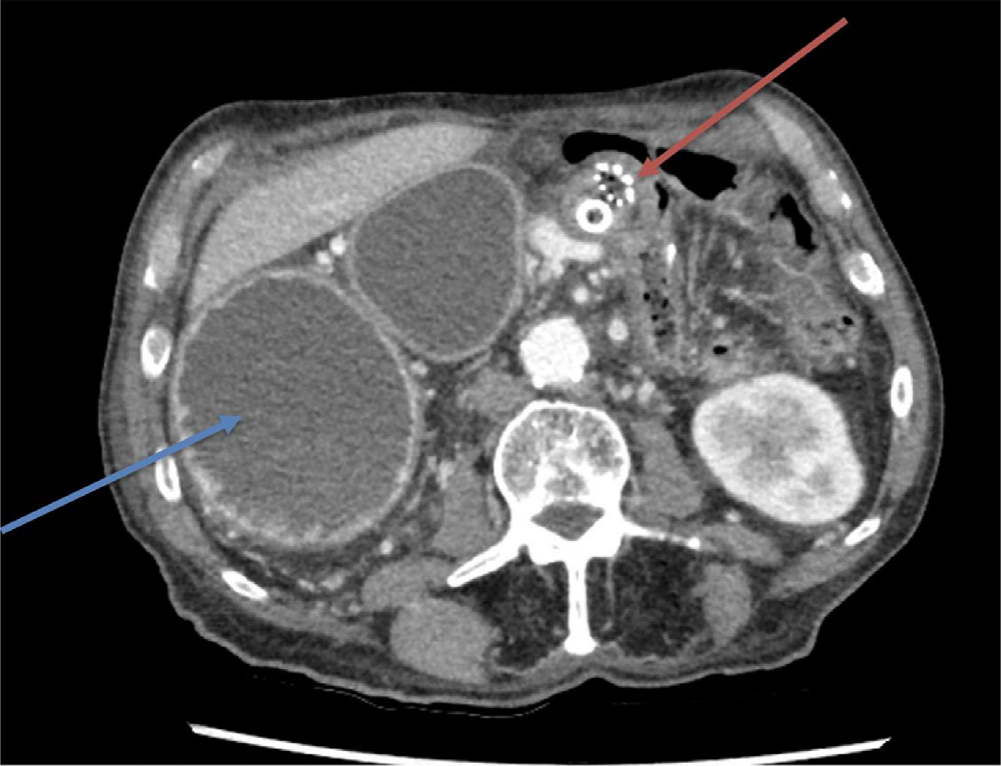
Axial slice of CT abdomen and pelvis with contrast demonstrating distended fluid-filled right-sided stomach. Red arrow - Compression of duodenal stent by soft tissue mass, likely relating to patient's history of cholangiocarcinoma. Blue arrow – Distended right-sided stomach.
